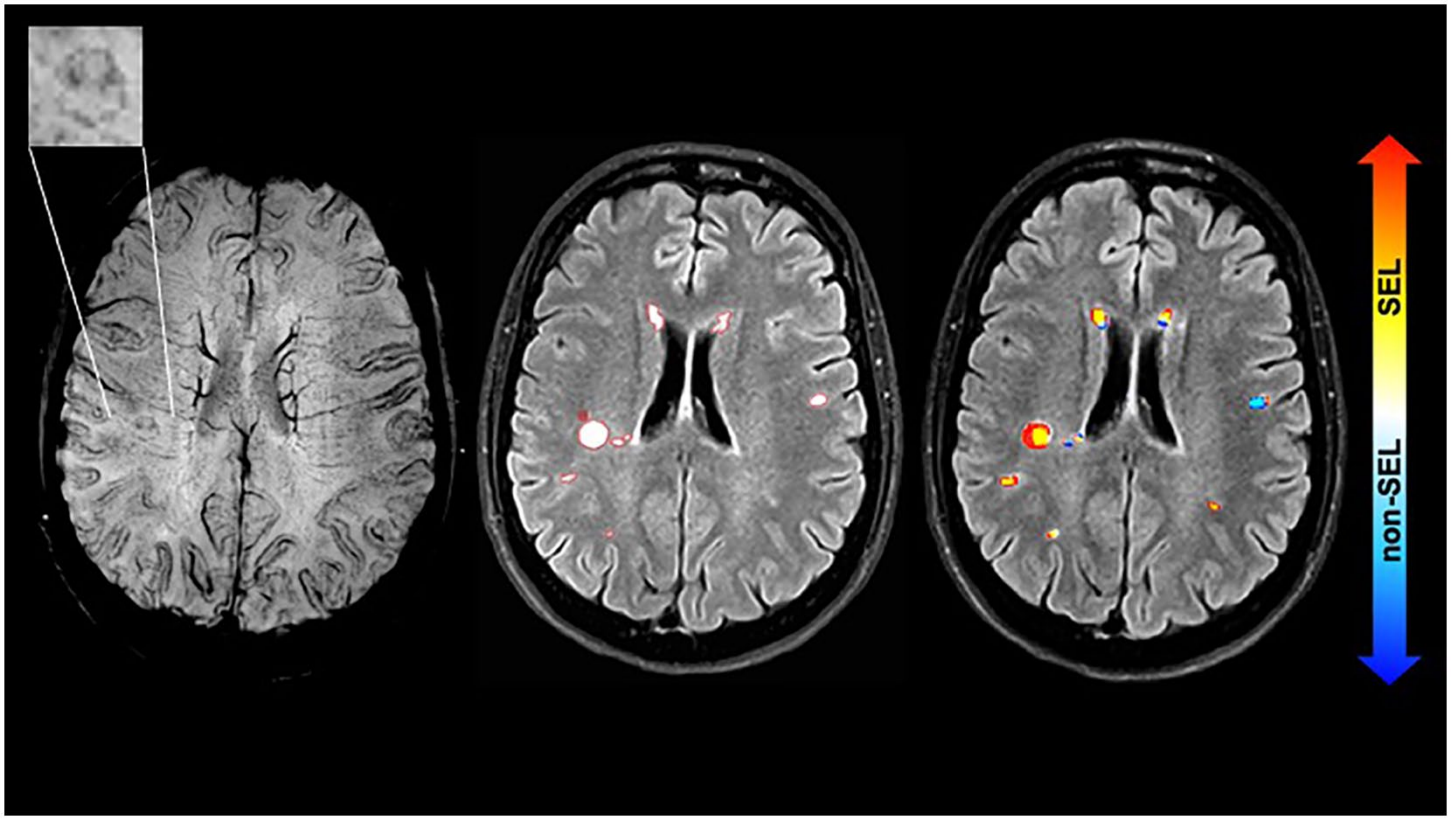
Example of a PRL at baseline and the correspondence to a SEL. The images show from left to right: SWI at baseline (the top left rectangle shows a magnification of the paramagnetic rim lesion), FLAIR with lesion mask countered in red, Jacobian map superimposed indicating that the PRL corresponds to a SEL. The images are from a 45-year-old person with a CIS and the MRI was performed 4 months after onset. At baseline the EDSS was 0 and last follow-up (~4 years) was 1.5. CIS: clinically isolated syndrome; EDSS: Expanded Disability Status Scale; PRL: paramagnetic rim lesion; FLAIR: fluid-attenuated inversion recovery; PRL: paramagnetic rim lesion; SWI: susceptibility-weighted imaging; SEL: slowly expanding lesion.
